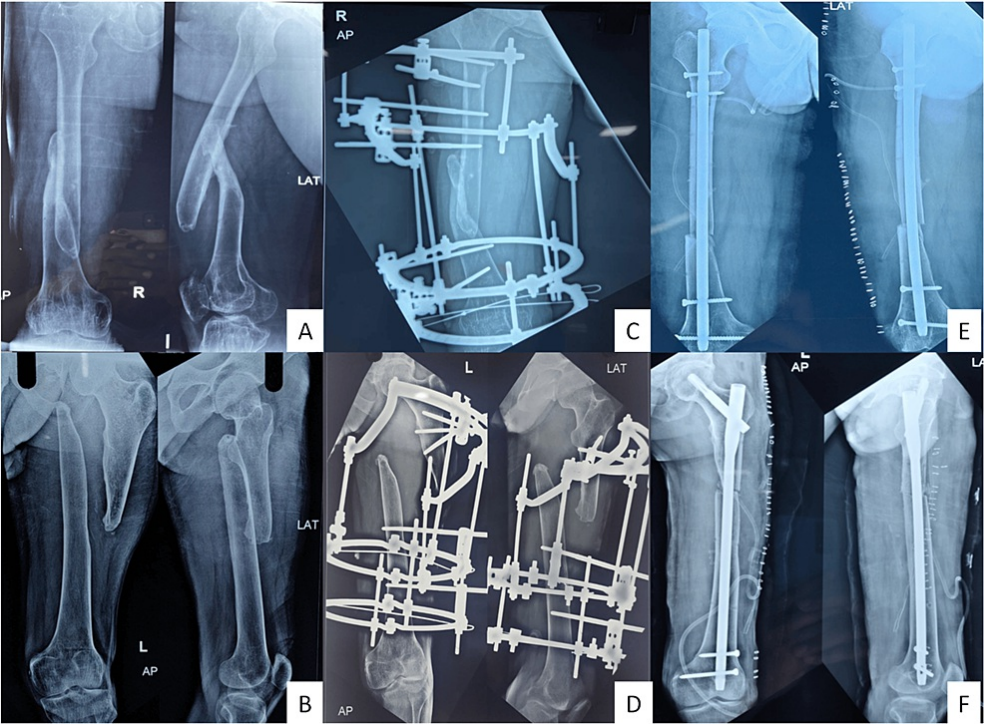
Preoperative (A, B) and postoperative radiograph with the application of the Ilizarov fixator for distraction (C, D), and following second-stage intramedullary nailing (E, F)
